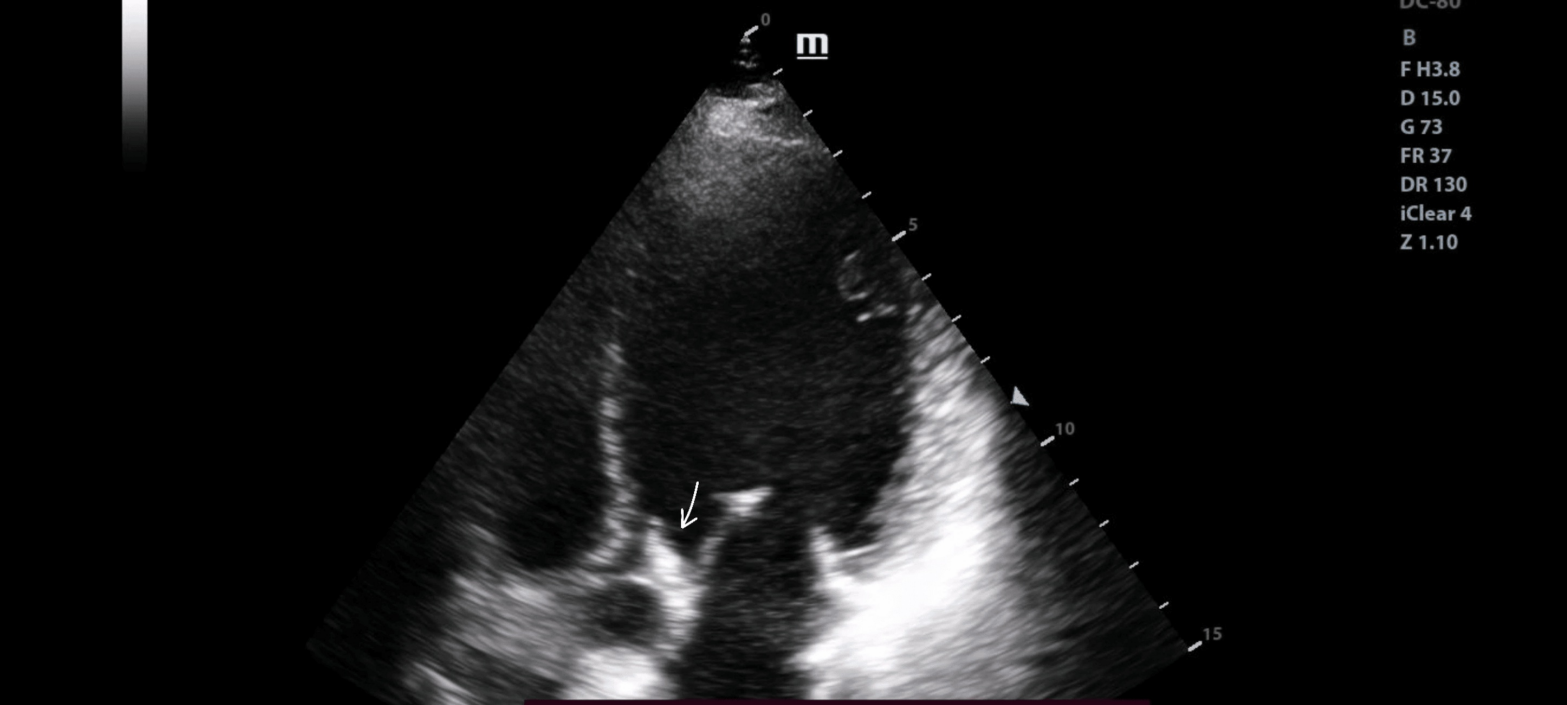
Echocardiogram on the day of admission. Normal chamber dimensions, no significant regional wall motion abnormality, adequate left ventricular systolic function, vegetation measuring 1.3 × 0.6 cm attached to the aortic valve, causing severe aortic regurgitation and no aortic stenosis, Grade 2 mitral regurgitation, mild tricuspid regurgitation, right ventricular systolic pressure of 26 mmHg plus right atrial pressure, no pulmonary artery hypotension. Good right ventricular function, patent foramen ovale present, intact interventricular septum, no pericardial effusion, inferior vena cava 1.5 cm collapsing with respiration, and ejection fraction of 50%.
